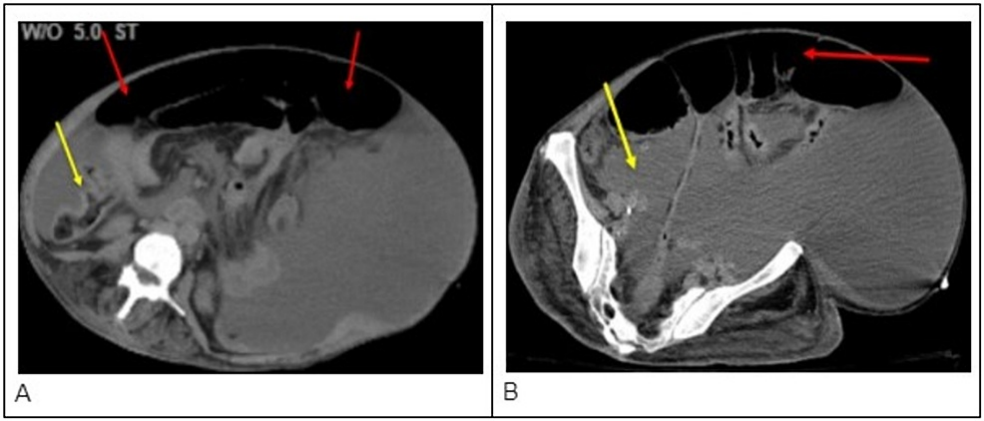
Two different CT scans of the abdomen taken during admission of this patient showing significant ascites (red arrows) and liver cirrhosis (yellow arrows)
